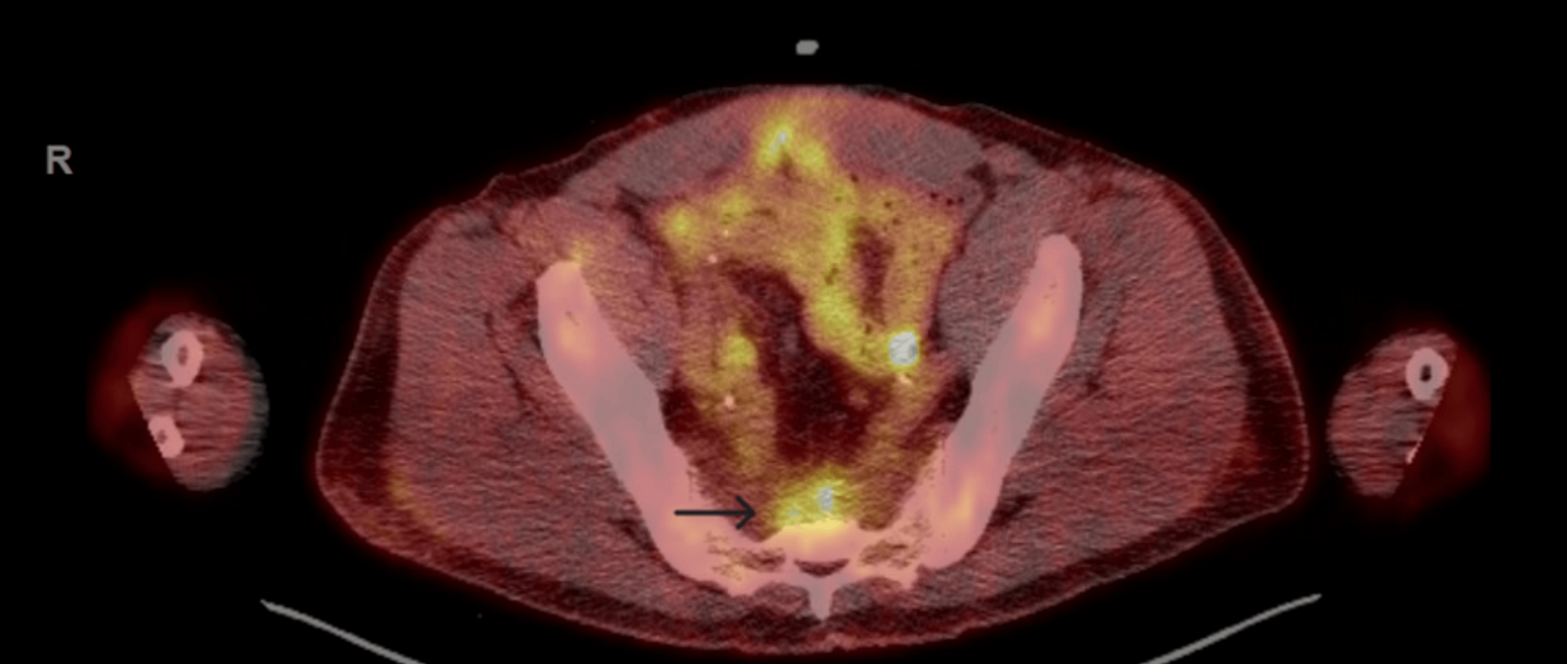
PET scan showing presacral mass-like opacity with intense increased radiotracer uptake, SUV max 7 SUV max: maximum standardized uptake value
